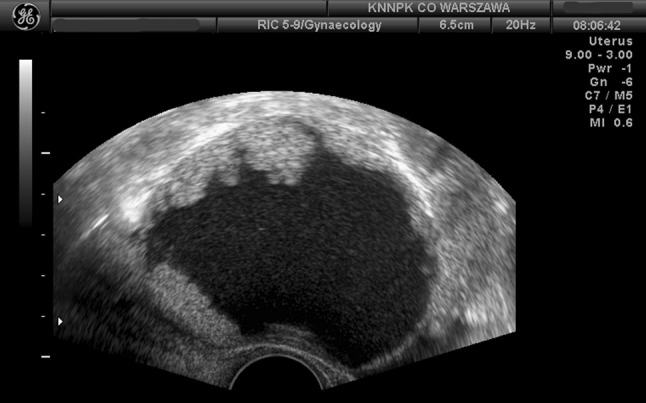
The characteristic ultrasonographic image of serous borderline tumor with typical endophytic outgrowth without free peritoneal fluid
